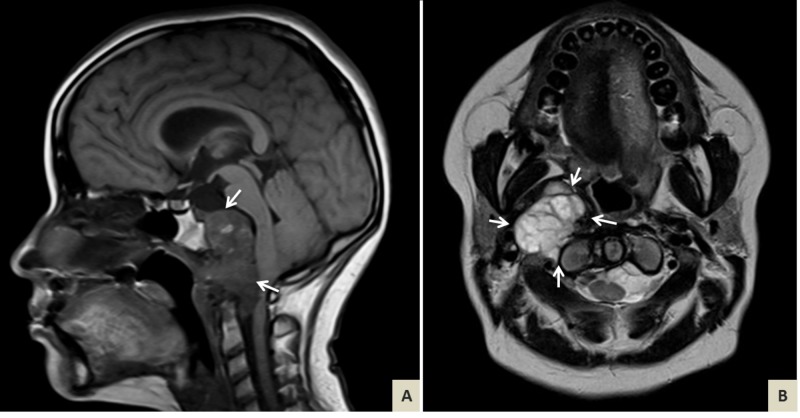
Brain magnetic resonance imaging (MRI) postsurgery. (A) T1-weighted sagittal and (B) T2-weighted axial MRI after partial excision show decompression of pons and medulla following reduction of tumor burden (white arrows).
